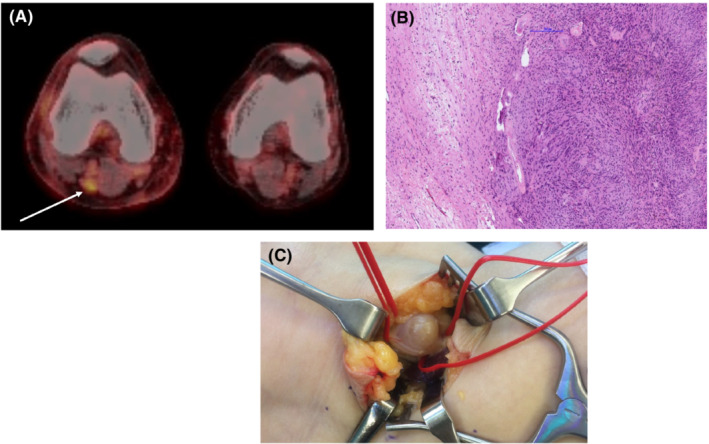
(A) FDG‐PET‐CT with high‐uptake right popliteal lesion. (B) Antoni A areas (hypercellular, with palisading) and Antoni B areas (hypocellular, myxoid) are characteristic for schwannoma. (C) Intraoperative suspicion of schwannoma
